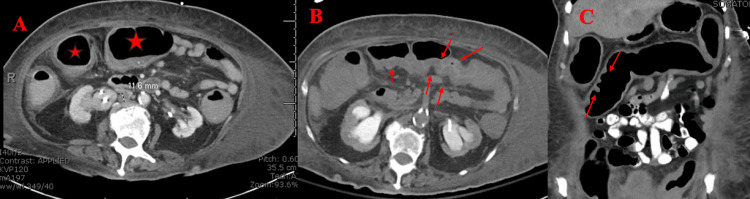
Abdominal CT scan A: Axial view with red stars indicating colonic dilation B: Axial view with red arrows indicating haustra effacement C: Sagittal view with red arrows indicating thumbprinting
